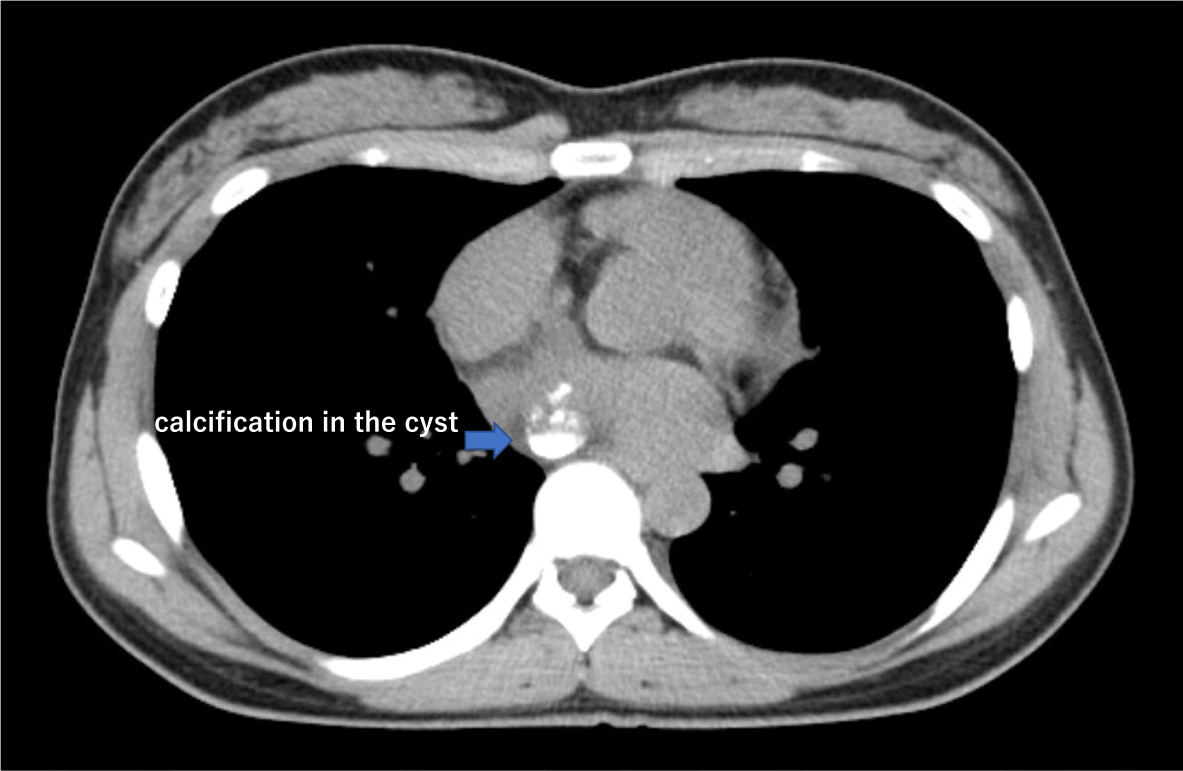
Preoperative CT scan indicating the calcification in the cyst
